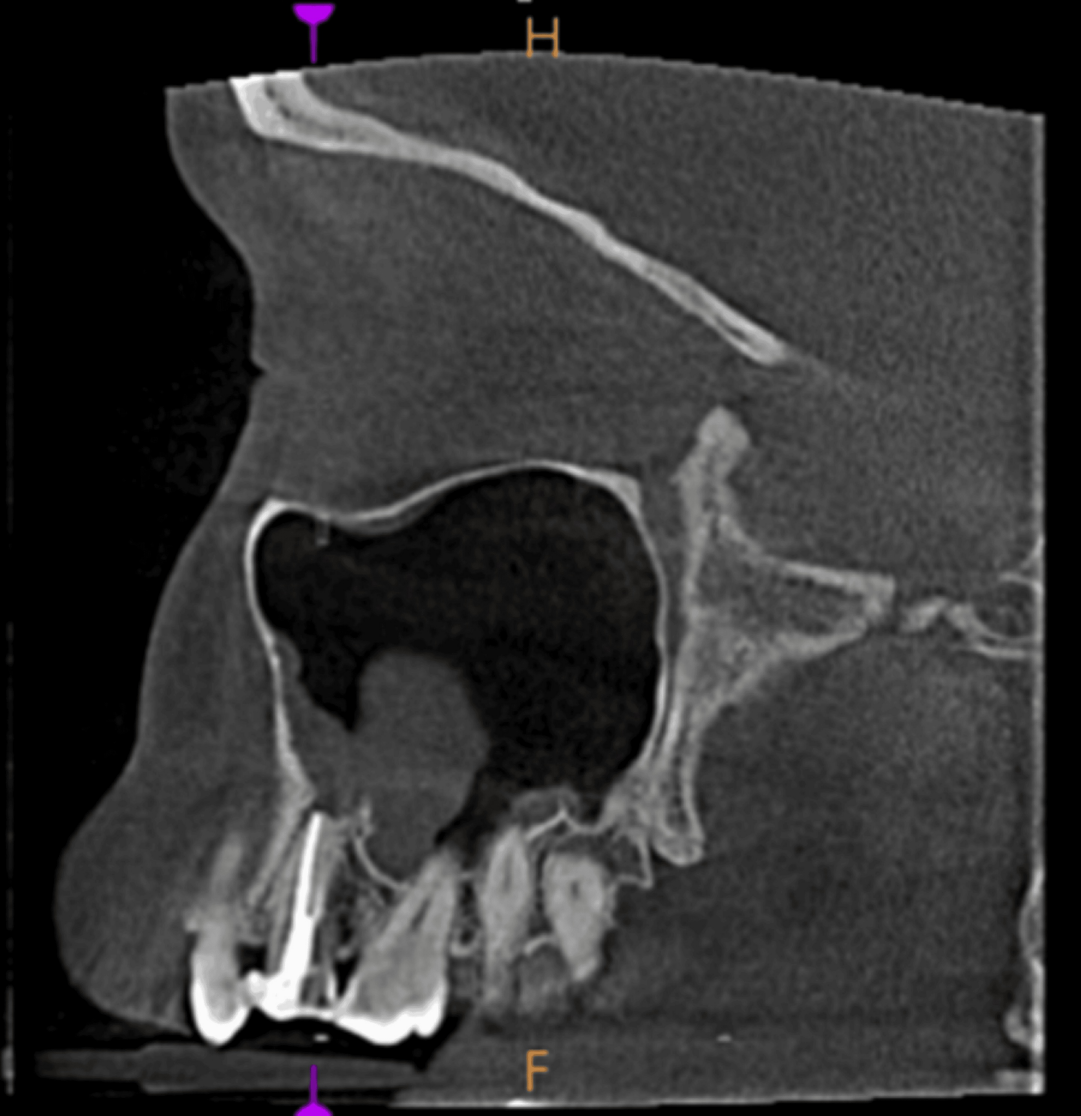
Periapical abscess in 15 with right maxillary sinus polypoidal thickening
